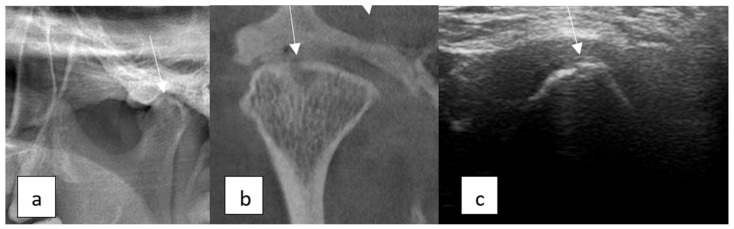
TMJ erosion: (a) panoramic radiography image, (b) CBCT image showing erosion in the superior surface of the condyle (sagittal view), and (c) USG image.
